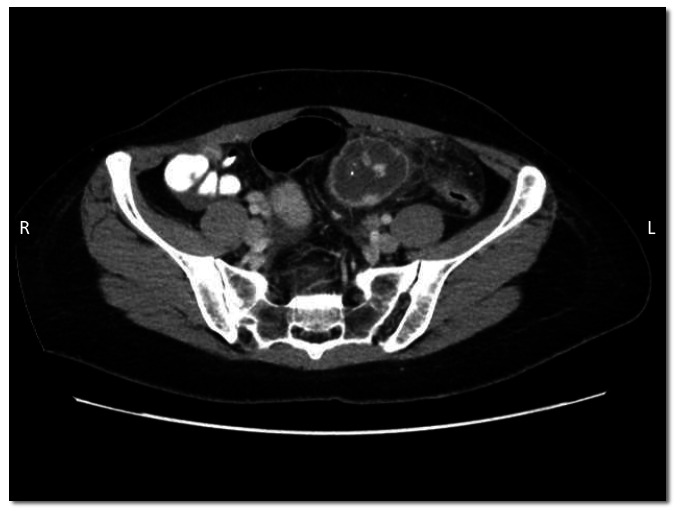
Axial-plane CT of the abdomen and pelvis demonstrating a hypodense lesion located left of the midline of the abdomen containing high-density material suggestive of hemorrhage. This lesion appeared inseparable from the small bowel and was surrounded by inflammatory changes. The adjacent colon showed mild thickening.
